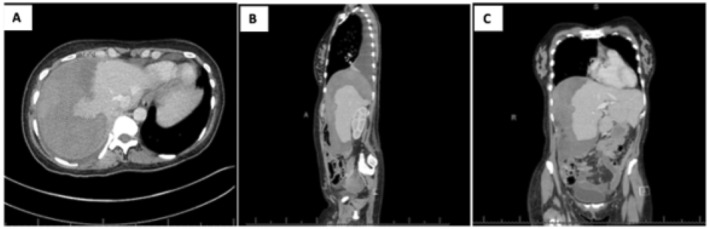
(A–C) Patient in Case 1, postoperative day 9. Abdominal CT scans demonstrate a perihepatic hematoma measuring 26 × 14 × 11 cm along with a large right pleural effusion. 1A. Transverse view; 1B. Sagittal view; 1C. Coronal view.
